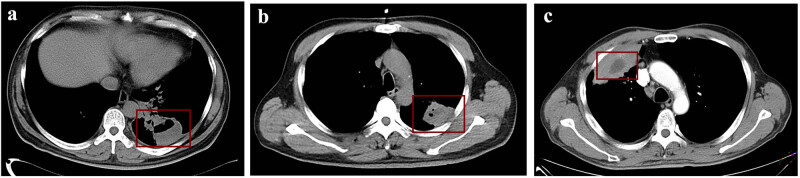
(a) Chest CT revealed cavitary lesion (56 mm × 41 mm) in the lower lobe of the left lung in case 1. (b) Chest-enhanced CT of case 2 showing a mass in the upper lobe of the left lung (about 45 × 34 mm). (c) A ches- enhanced CT scan revealed a soft tissue mass shadow in the upper lobe of the left lung in case 3.
